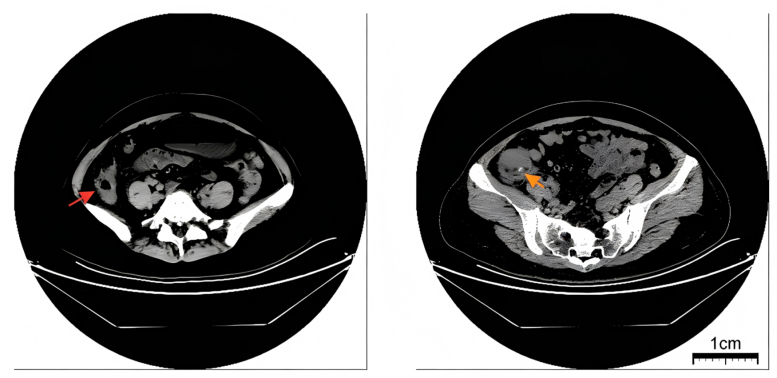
CT imagings (The red arrow indicates diverticulum, and the orange arrow indicates fecal stones). CT = computed tomography.
